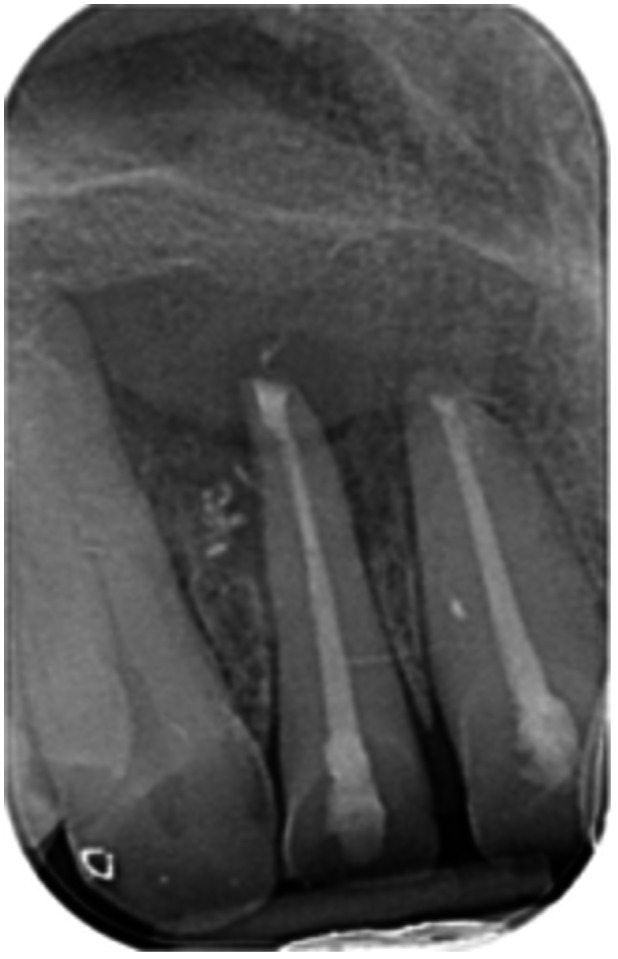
Case 6: The preoperative CBCT and periapical radiograph show an extensive periapical radiolucency associated with teeth 11 and 12, which has extended to tooth 13 as well. Apical surgery was performed on teeth 11 and 12 only, as tooth 13 was deemed vital. At 6 months, marked healing can be seen, evidenced by a reduction in the periapical radiolucency size associated with teeth 11 and 12, with PDL reattachment noted around the root of tooth 11.
